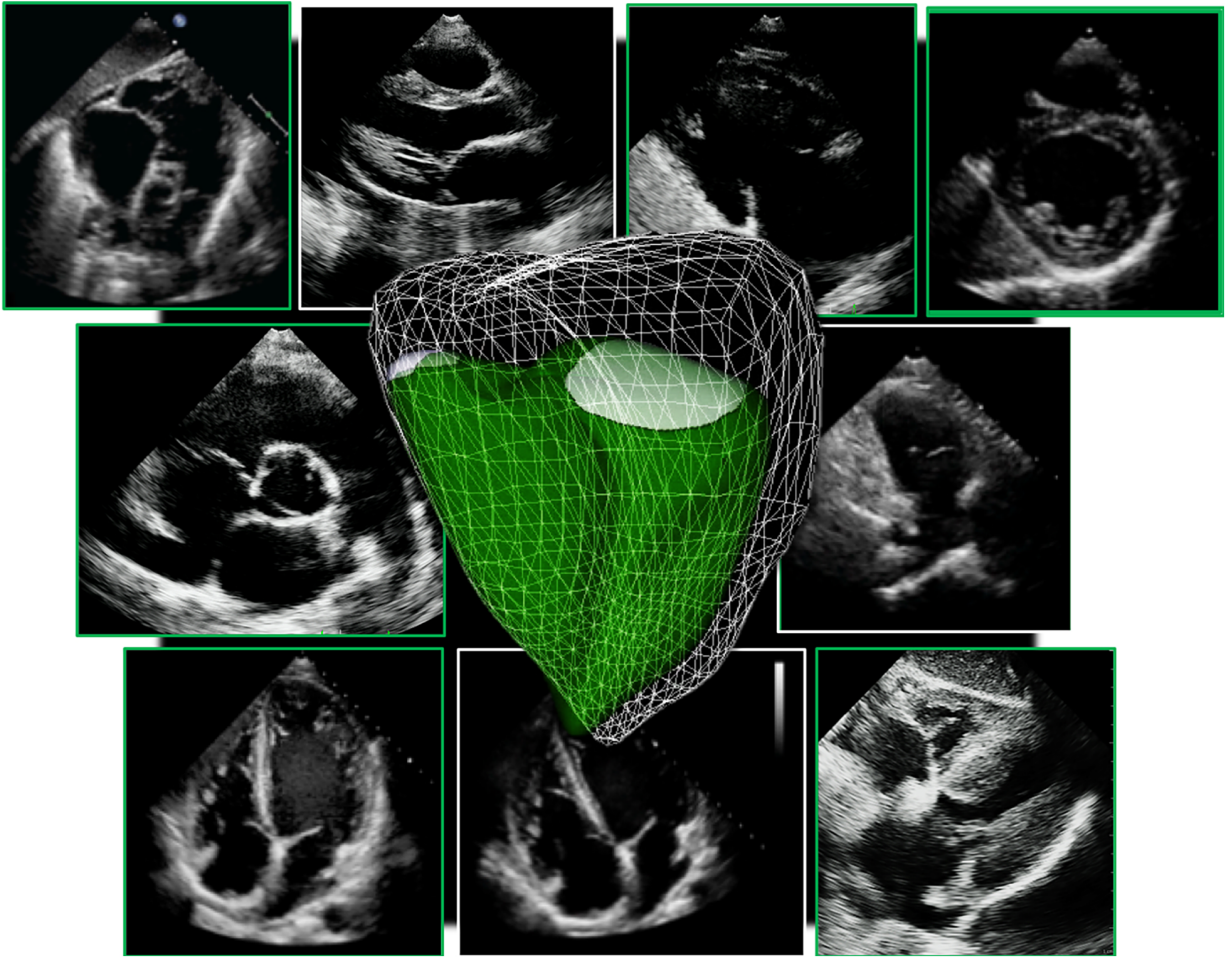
Collection of all 2D standard imaging planes used on routine transthoracic echocardiography to assess the right ventricle. A 3D right ventricle endocardial surface is superimposed on top (in green).
